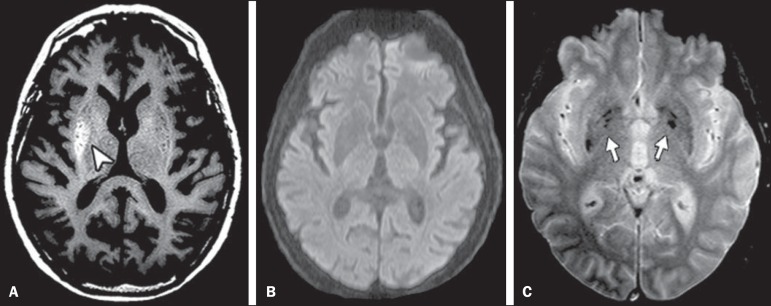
MRI findings two weeks after the initial presentation. A:
